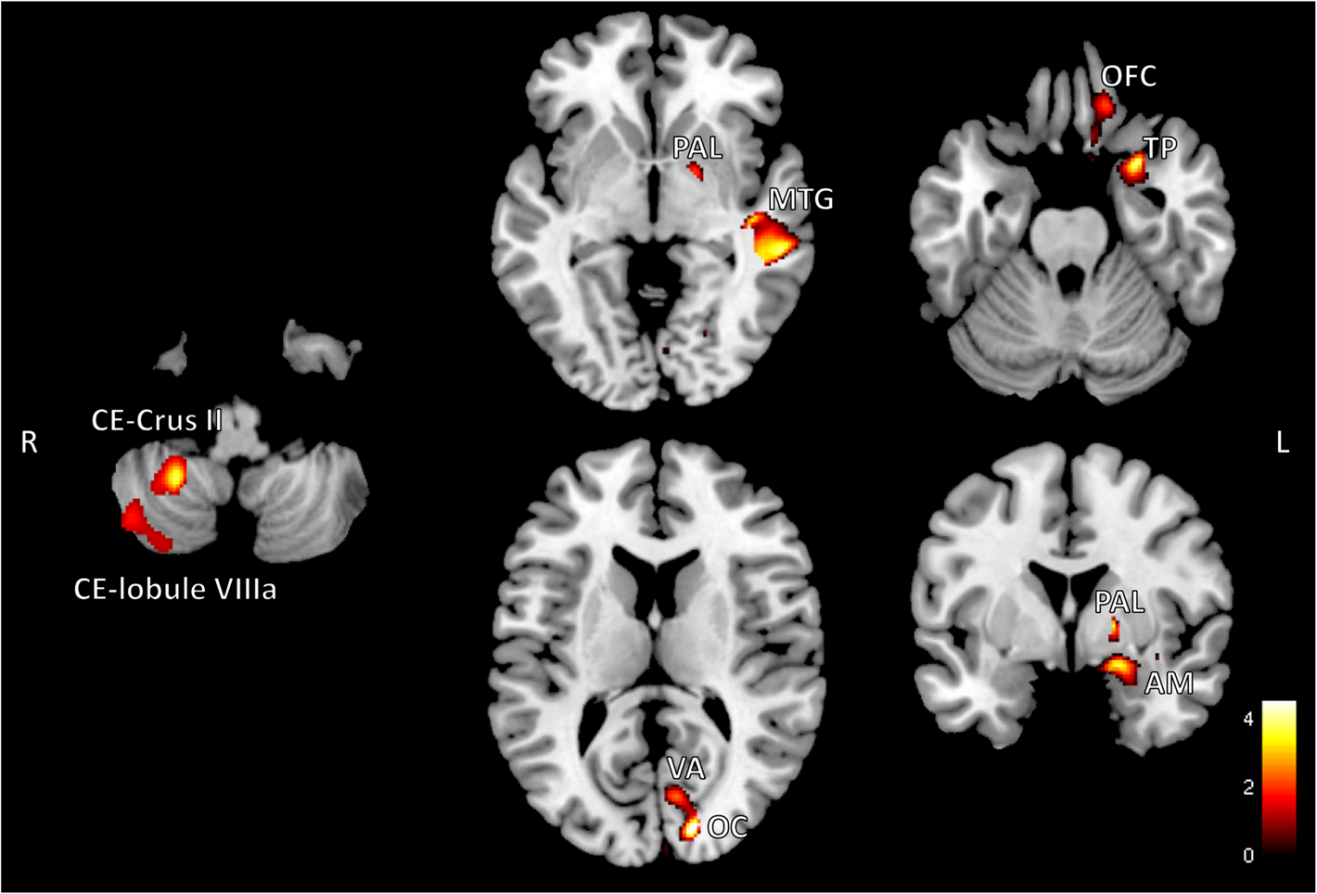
SPM regions superimposed on a high-resolution T1-weighted scan show decreased GM volume in patients with CM compared to HCs (*p* < 0.005 uncorrected). Areas with significantly reduced GM volume are observed in the cerebellum (CE), left primary occipital cortex (OC), visual association area (VA), left middle temporal gyrus (MTG), left amygdala (AM), left temporal pole (TP), left pallidum (PAL), and left orbitofrontal cortex (OFC). Labels of the cerebellum stem from the Spatially Unbiased Infraorbital Template atlas. L = left, R = right
